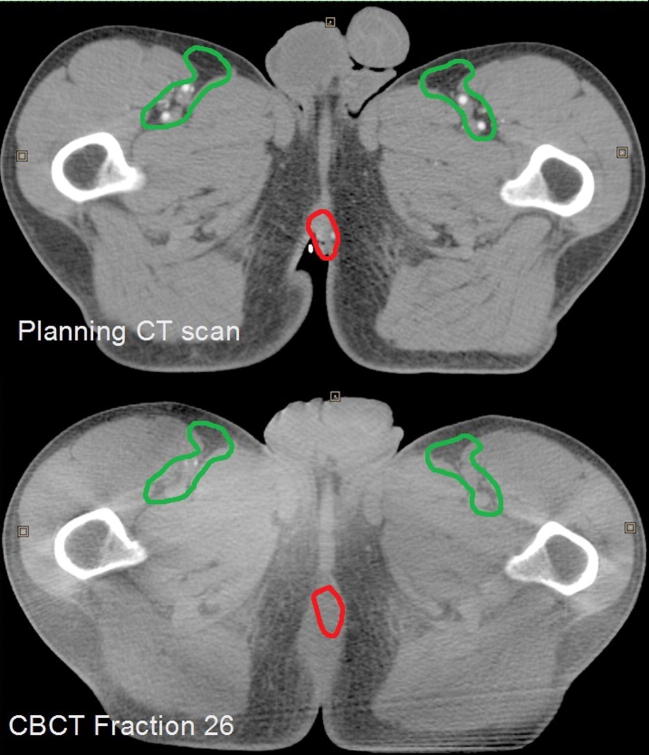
Patient where the CBCT pelvic bony auto-match resulted in good alignment of the inguinal target (green contour) but poor alignment of anal target (red contour). (For interpretation of the references to colour in this figure legend, the reader is referred to the web version of this article.)
